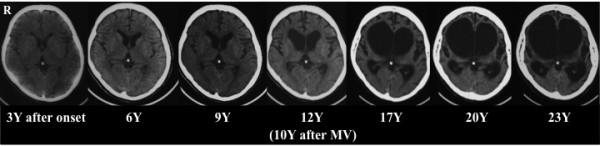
Computed tomography scans of the older sibling. At the age of 45 years (12 years after amyotrophic lateral sclerosis onset; 10 years after the initiation of mechanical ventilation), the scan shows marked brain atrophy. After the subsequent 10 years, whole brain atrophy had markedly progressed. Y-Years; MV-mechanical ventilation.
